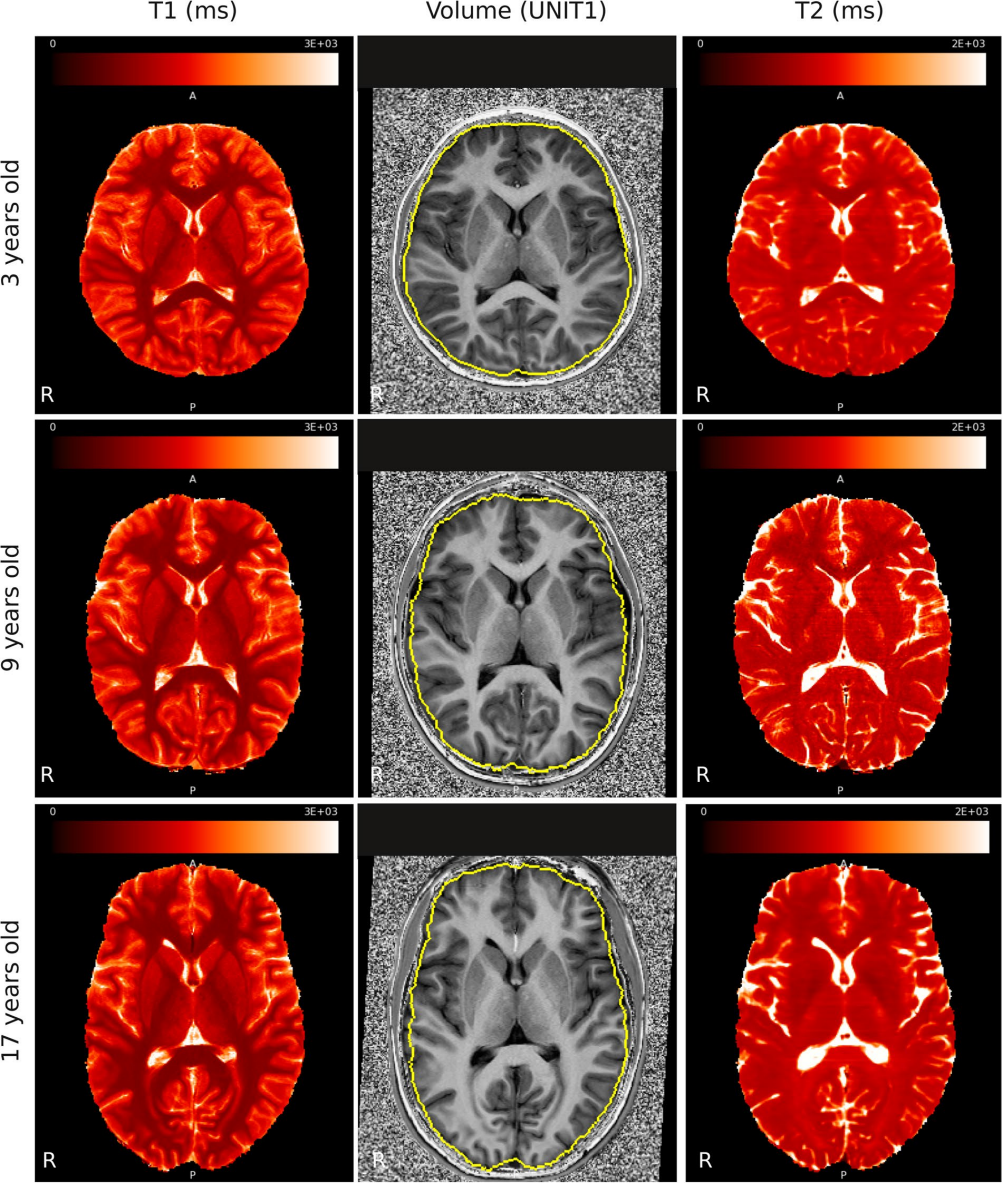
Representative scans. T1 map with relaxation time in ms (left), UNI T1-weighted (center) and T2 map with relaxation time in ms (right), scans for 3 years old (up, subject sub-HVHRIV), 9 years old (middle, subject sub-LIDPOT) and 17 years old (bottom, subject sub-MKP611) subjects are shown. Brain segmentation from our in-house software is depicted as a yellow outline on UNI images. Brain T1 and T2 maps are shown in red, overlaid on greyscale renderings of the raw maps. Data acquisition and quality were high for a pediatric population.
